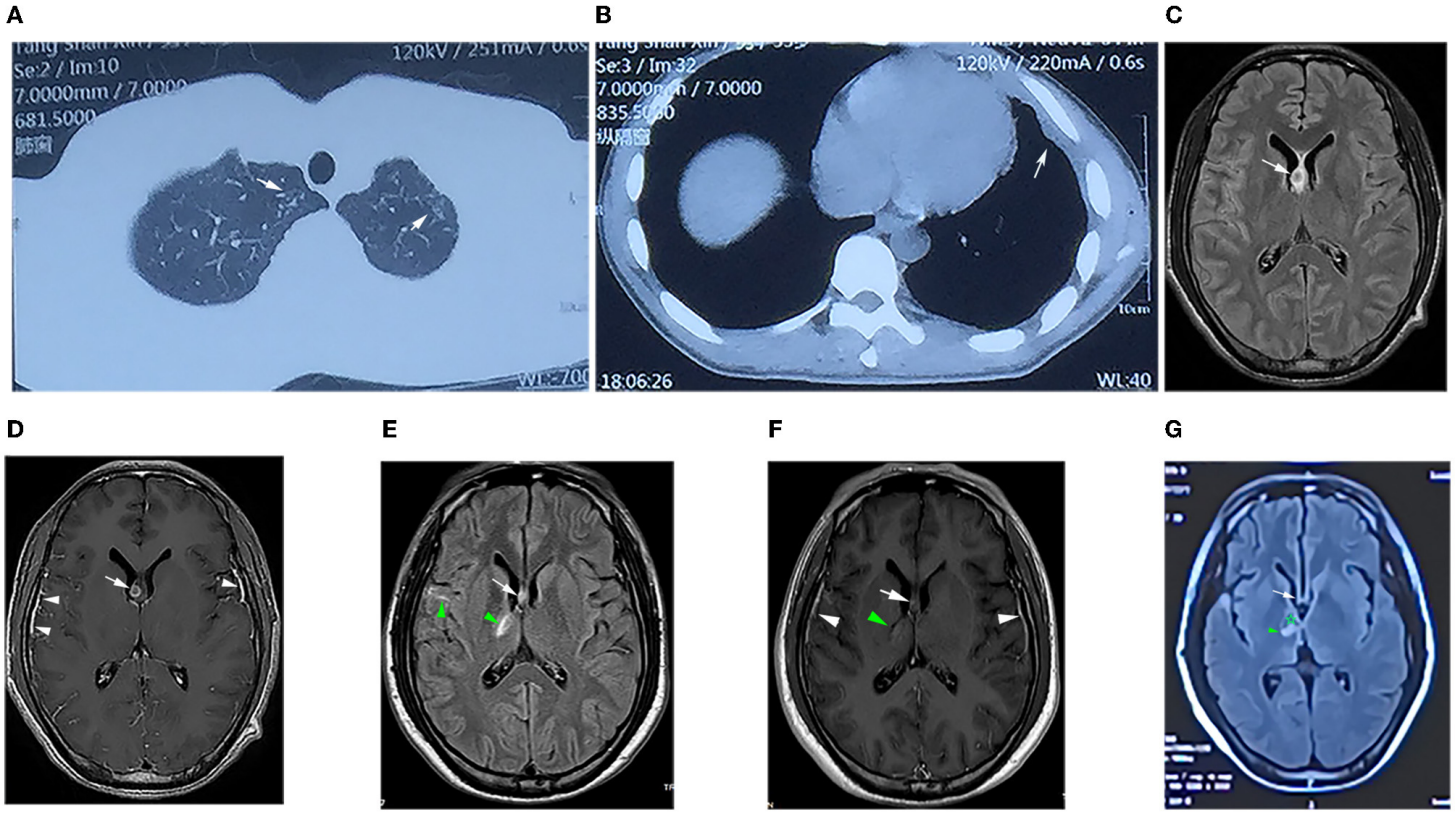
(A) Computed tomography (CT) of the chest showing patchy high-density shadows in the upper lobes of both lungs (white arrow), suggesting secondary to tuberculosis. (B) CT scan revealed thickening of the left pleura and encapsulated effusion (white arrow). (C) T2-weighted magnetic resonance imaging (T2WI) FLAIR showing small circular isointense, slightly long hyperintense in the right lateral ventricle subependymal (white arrows). (D) Contrast-enhanced T1WI showing that the lesion was ring-enhancing (white arrows) and significant enhancement of the bilateral temporal leptomeningeal (white triangle). (E) T2WI FLAIR image showing that the right subependymal lesion (arrow) was smaller than that in (C), and the right basal ganglia and right temporal lobe were patchy and line-like hyperintensity (green triangles). (F) Contrast-enhanced T1WI showing that the lesion in the right subependymal was smaller than that in (D) (white arrow), the lesion in the right basal ganglia was slightly enhanced (green triangle), and the meninges were thicker as before (white triangle). (G) T2WI FLAIR image showing that the subependymal lesion had basically disappeared (white arrow), the right basal ganglia lesion was slightly smaller (green triangle), and softening could be seen inside (✰).
